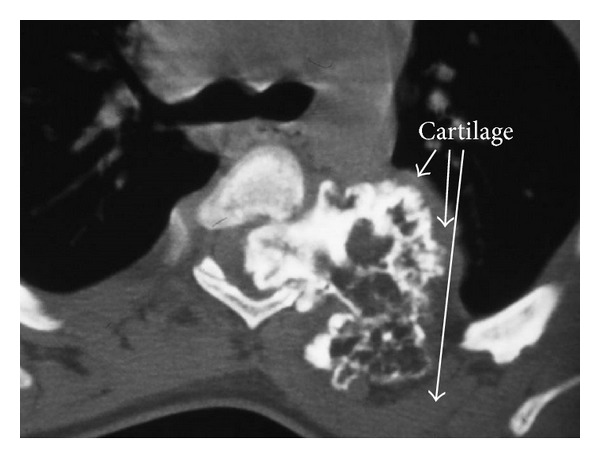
Axial CT of T3 scan showed the extent of the involvement of the left lamina and compression of the cord. The cauliflower appearance corresponds to the ossified matrix of the osteochondroma (huge intraspinal mass with heterogeneous density). This ossified matrix is surrounded by cartilage tissue, the osseous tumour originating from the left T3–5 facet joint (arrows).
